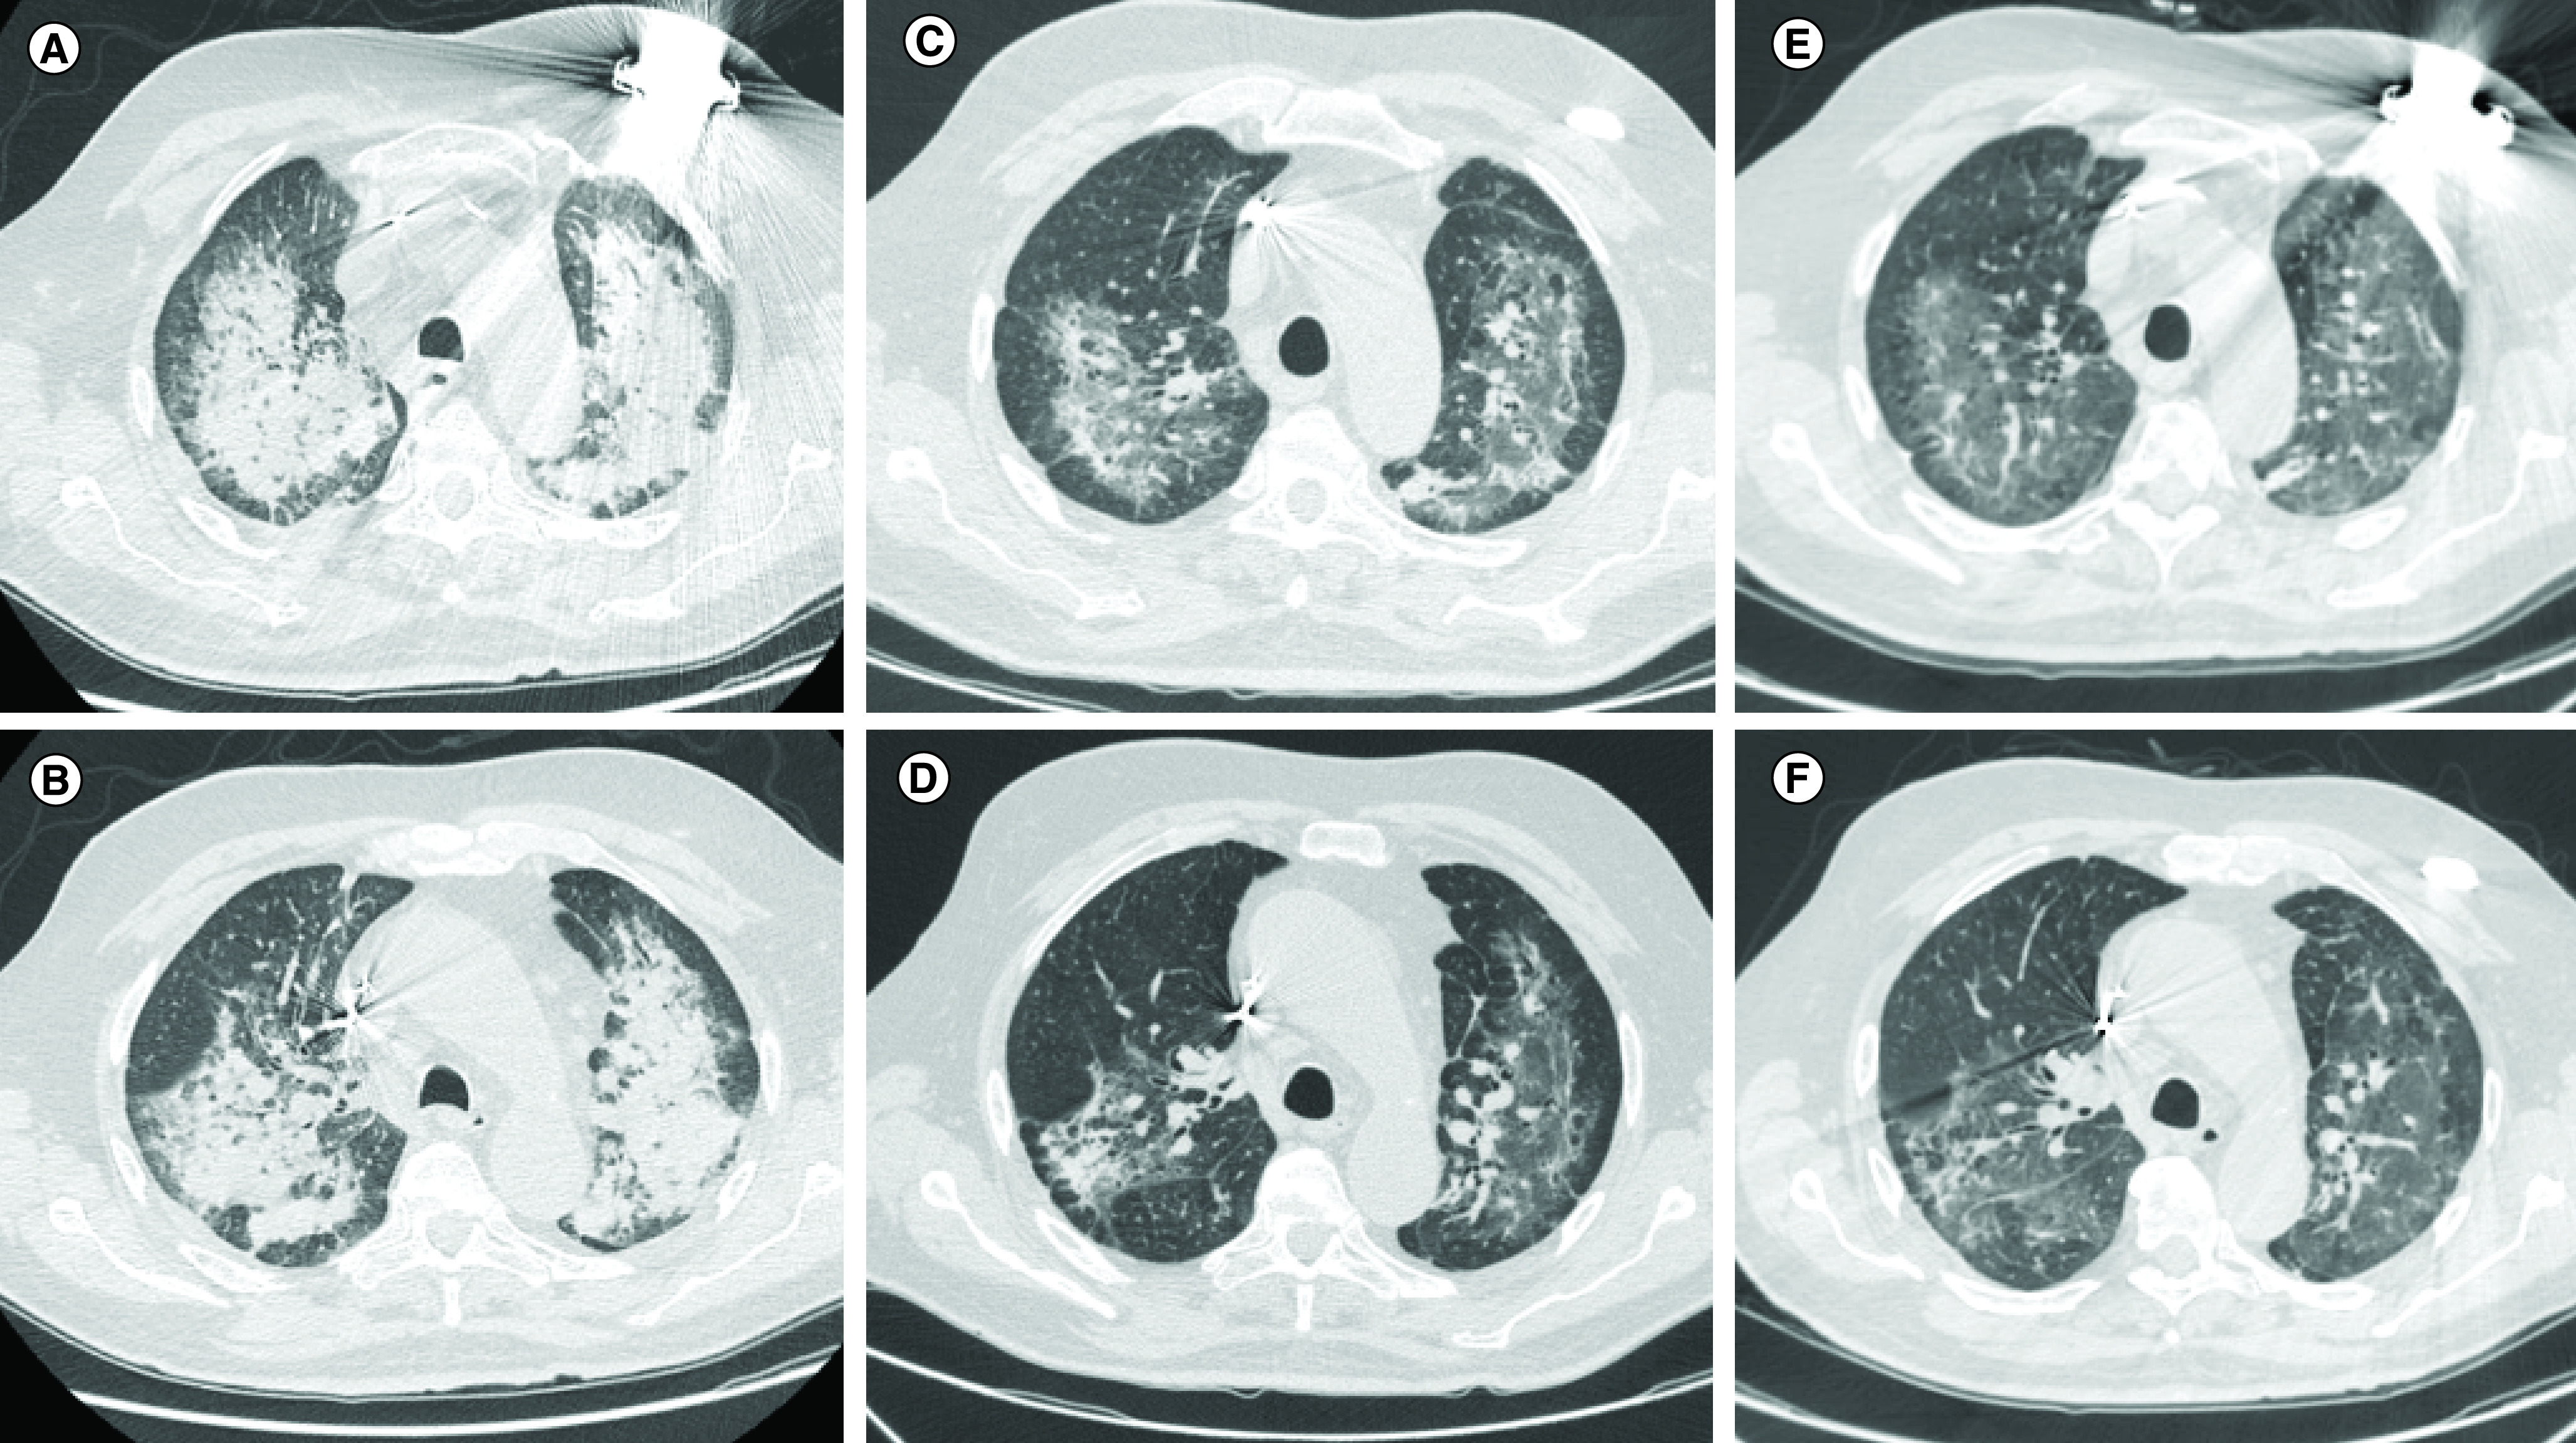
High-resolution computed tomography of a 79-year-old man with amiodarone induced organizing pneumonia. Extended multifocal parenchymal thickening at the (A) apical and (B) lower lobes, bilaterally, with vast ground glass areas and pseudonodular parenchymal consolidations. Progressive resolution of organizing pneumonia after 2 months (C & D) and after 3 months of steroid therapy and drug interruption (E & F), with persisting ‘ground glass’ areas associated with fibrotic-cicatricial manifestations, such as retractions of costal pleural sheets, mostly in lower lobes.
